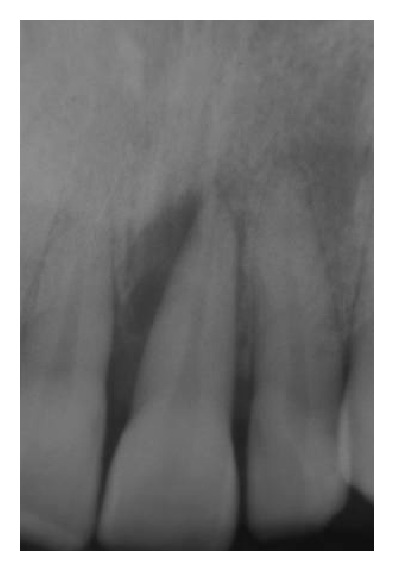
Periapical X-ray at baseline.
